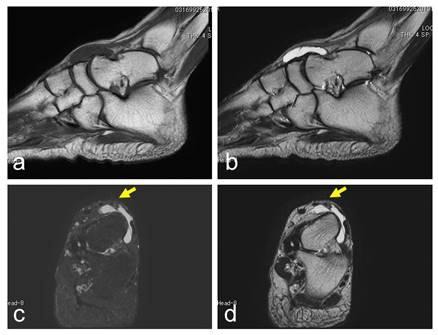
A 55-year-old female with a ganglion in the dorsal foot. A cystic lesion, with low signal intensity on a T1-weighted image (a) and high signal intensity on a T2-weighted image (b–d) located over the talus to the navicular (a–b). The dorsalis pedis artery is located over the lesion (yellow arrows; c–d). A T2-weighted image with fat-suppression (c). Sagittal views (a–b) and axial views (c–d).
